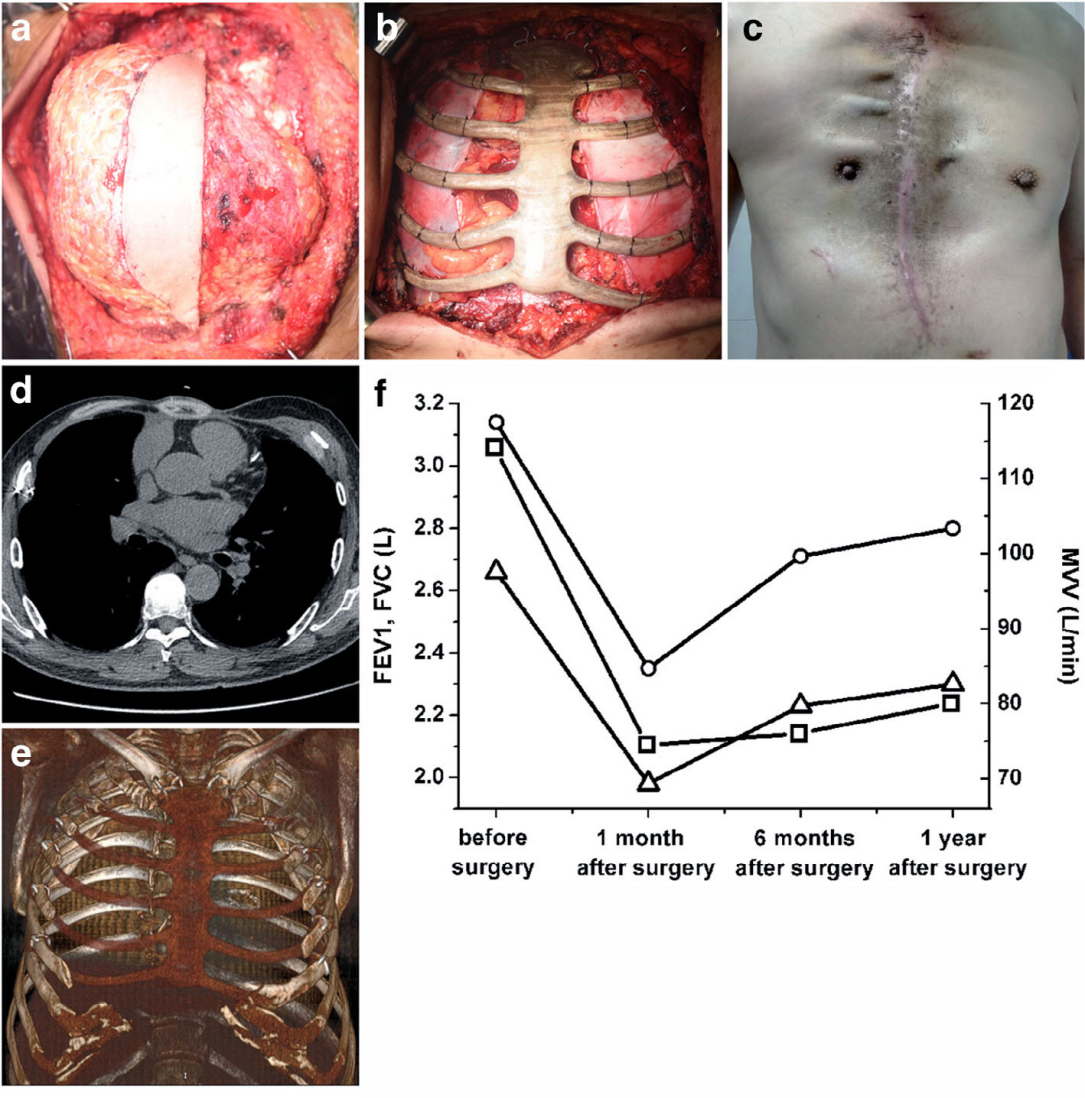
(a) The tumor was seen to be invading the sternum, ribs, proximal pleura and muscle. The whole tumor was exposed and part of the skin was left on the surface of the tumor, which needed to be removed with the tumor because it was less than 2 cm away from it. (b) A complete en bloc resection of the anterior chest wall was performed and the 3DP polyether‐ether‐ketone (PEEK) implant was anchored to the clavicle and remaining ribs using steel wires. A pericardial patch was densely suspended on the inner surface of the PEEK implant. (c) The incision site of the patient one year after surgery. (d) Chest computed tomography (CT) scan of the patient one year after surgery. (e) Three‐dimensional reconstruction of chest CT images of the patient one year after surgery. (f) The changes in pulmonary function values pre‐ and postoperatively, including
